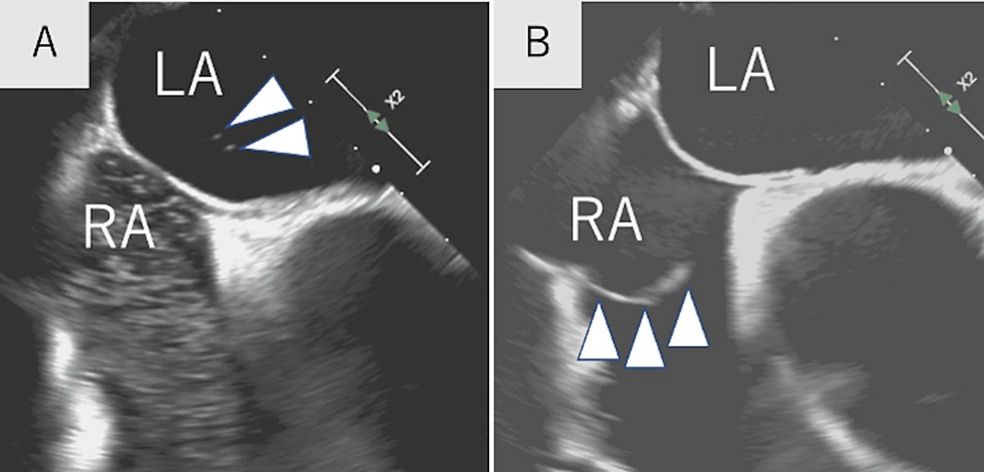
RLS and a large Eustachian valve on transesophageal echocardiography A: Microbubble test showed RLS (arrowheads). B: A large Eustachian valve was found in the right atrium (arrowheads). LA, left atrium; RA, right atrium; RLS, right-to-left shunt
